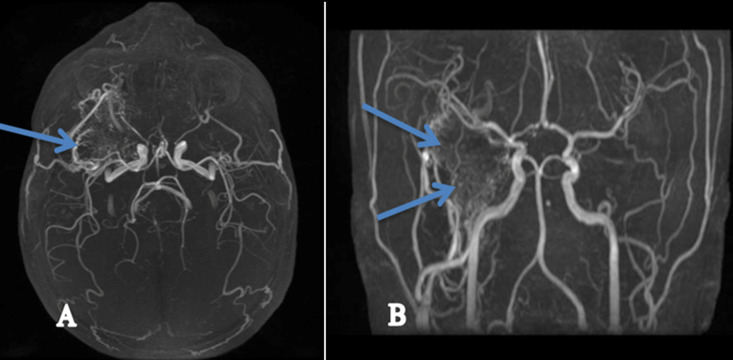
the patient’s angio-MRI showing the tumour blush and feeder pedicles, indicating the hyper-vascularization of this tumour (the angiofibroma) as shown by the blue arrows
